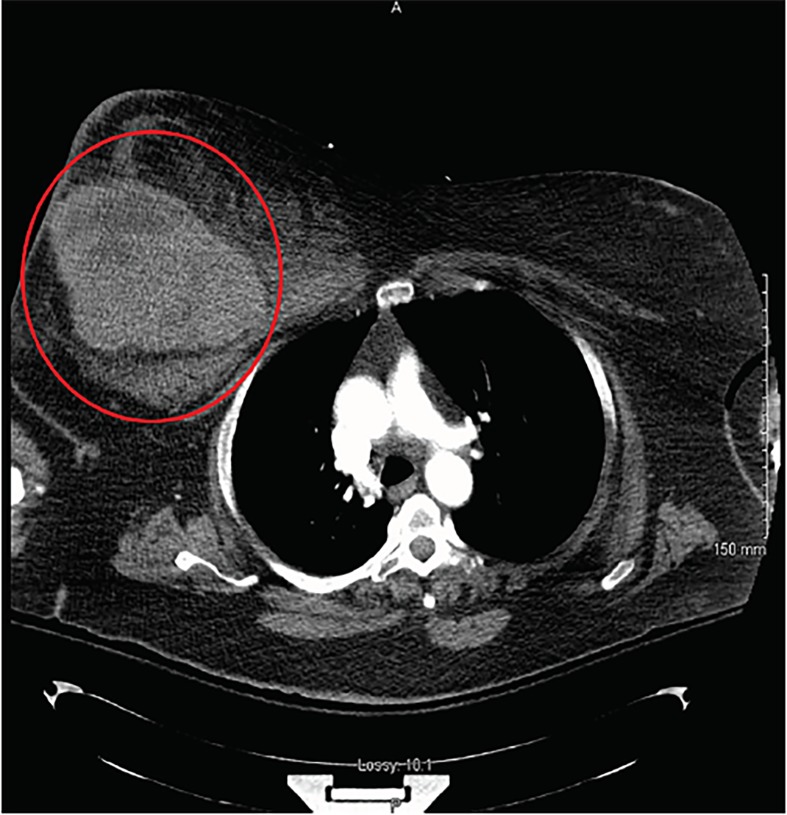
Axial view of a computed tomography of the chest revealing large right breast hematoma (red circle).
